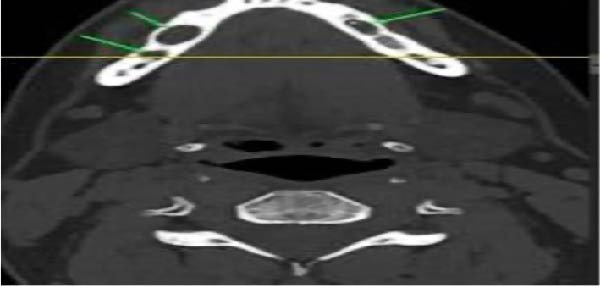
Preoperative computerized tomography (CT) scan (axial cut) revealed bilateral radiolucenct lesions.
